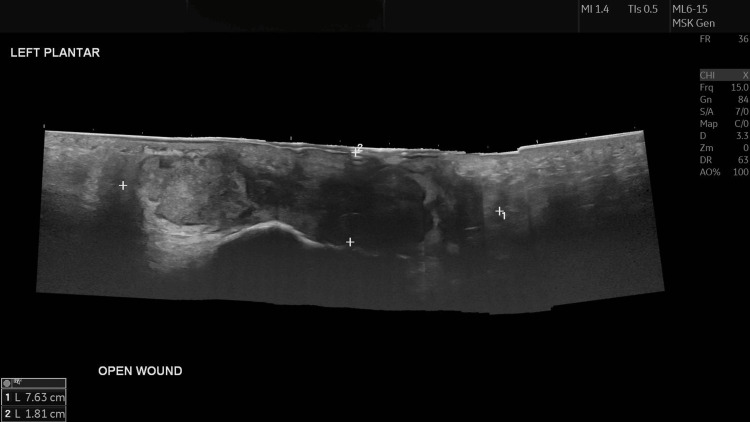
Ultrasound of the left foot demonstrating a 7.6 cm × 1.8 cm × 2.5 cm heterogeneous collection
